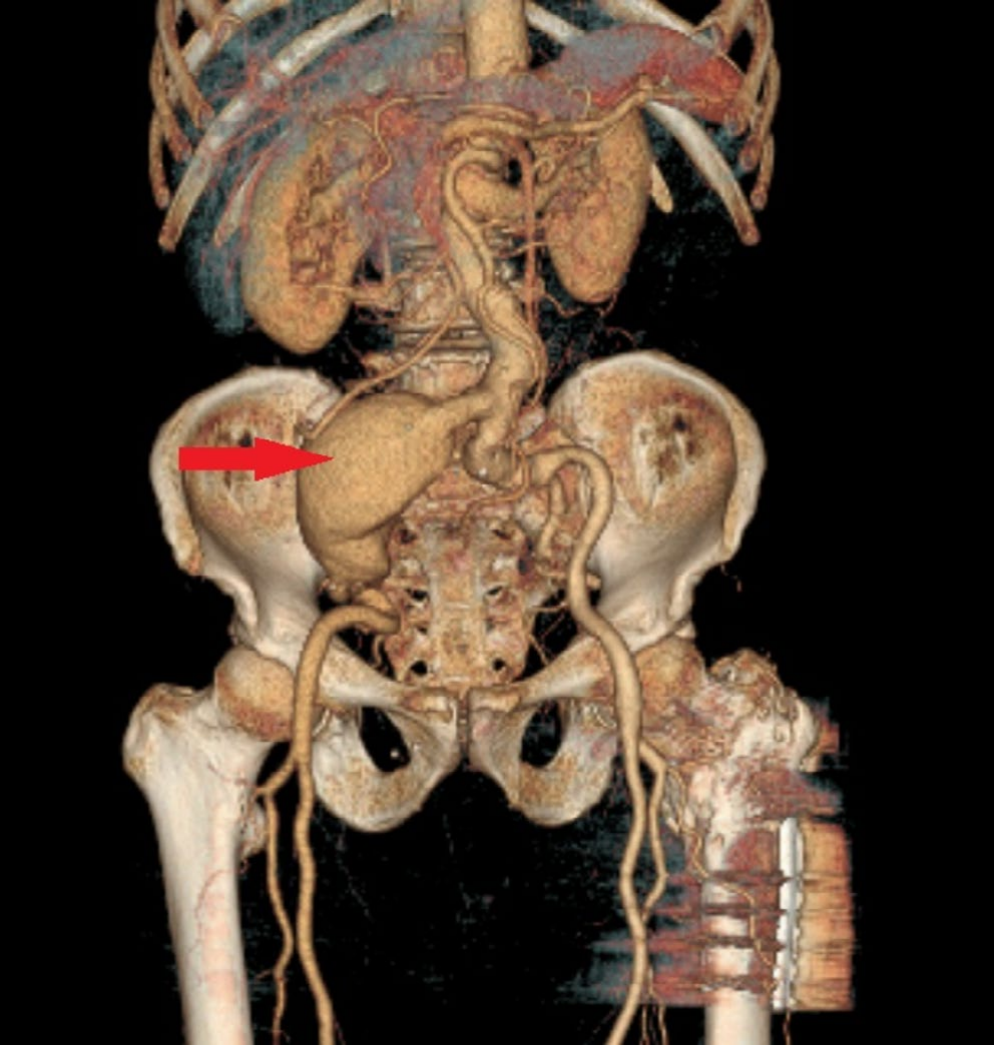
A red arrow indicates the Right common iliac artery aneurysm in a 3D CTA view.
